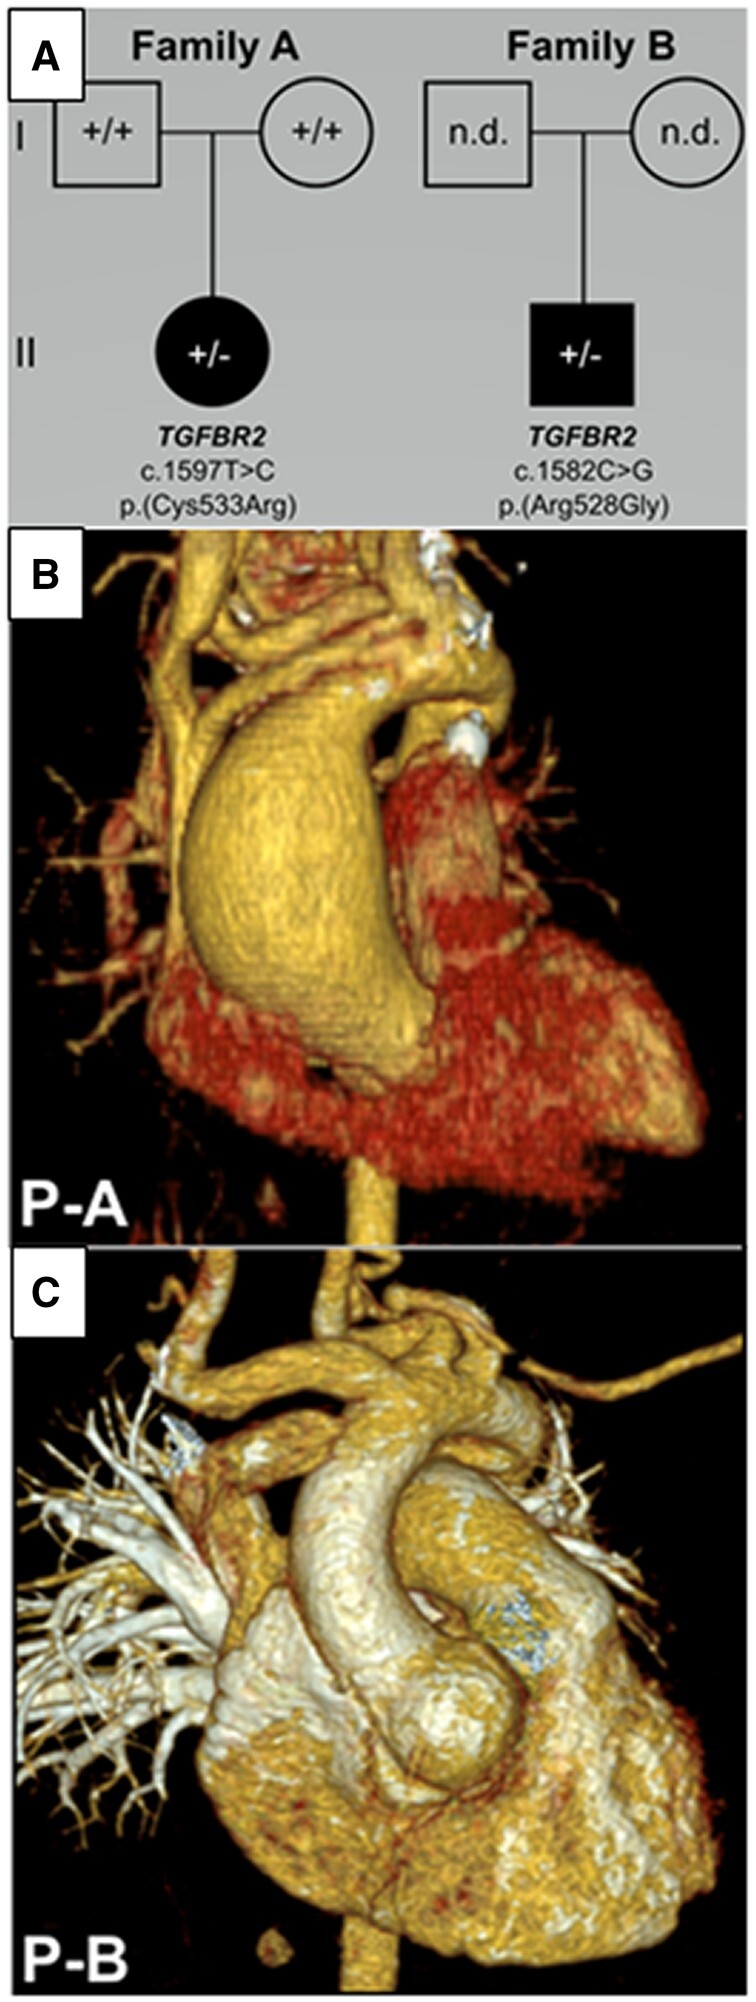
(A) Family tree of the 2 patients. (B) Preoperative 3-dimensional computed tomography of the suprajunctional ascending aortic aneurysm of patient A. (C) Preoperative 3-dimensional computed tomography of the aortic root aneurysm of patient B.
